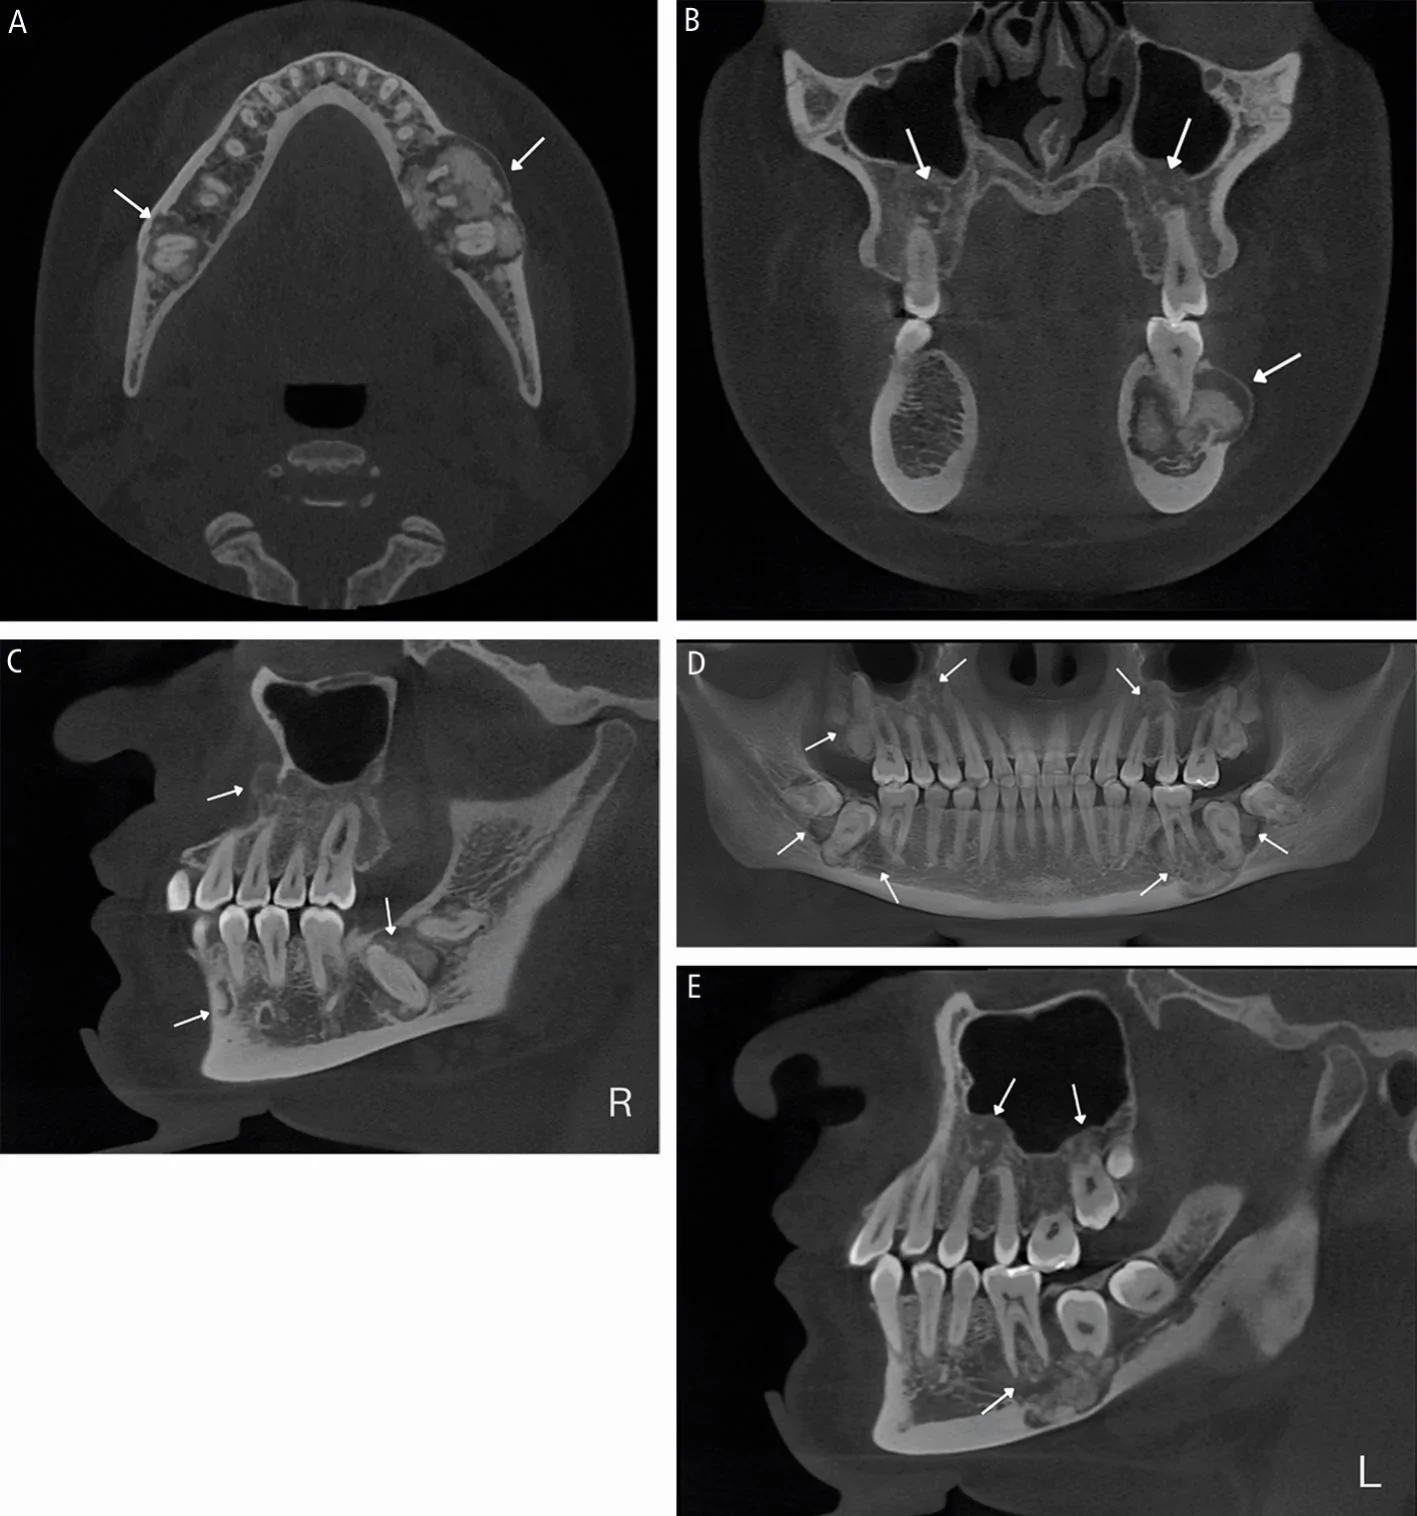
Axial (A), coronal (B), sagittal (C and E), and panoramic (D) CBCT reconstructions of Case 2 at 23 years of age, demonstrating mixed-density lesions (arrows) and unerupted teeth in all four quadrants, with bone expansion in the maxilla bilaterally and in the left mandible
